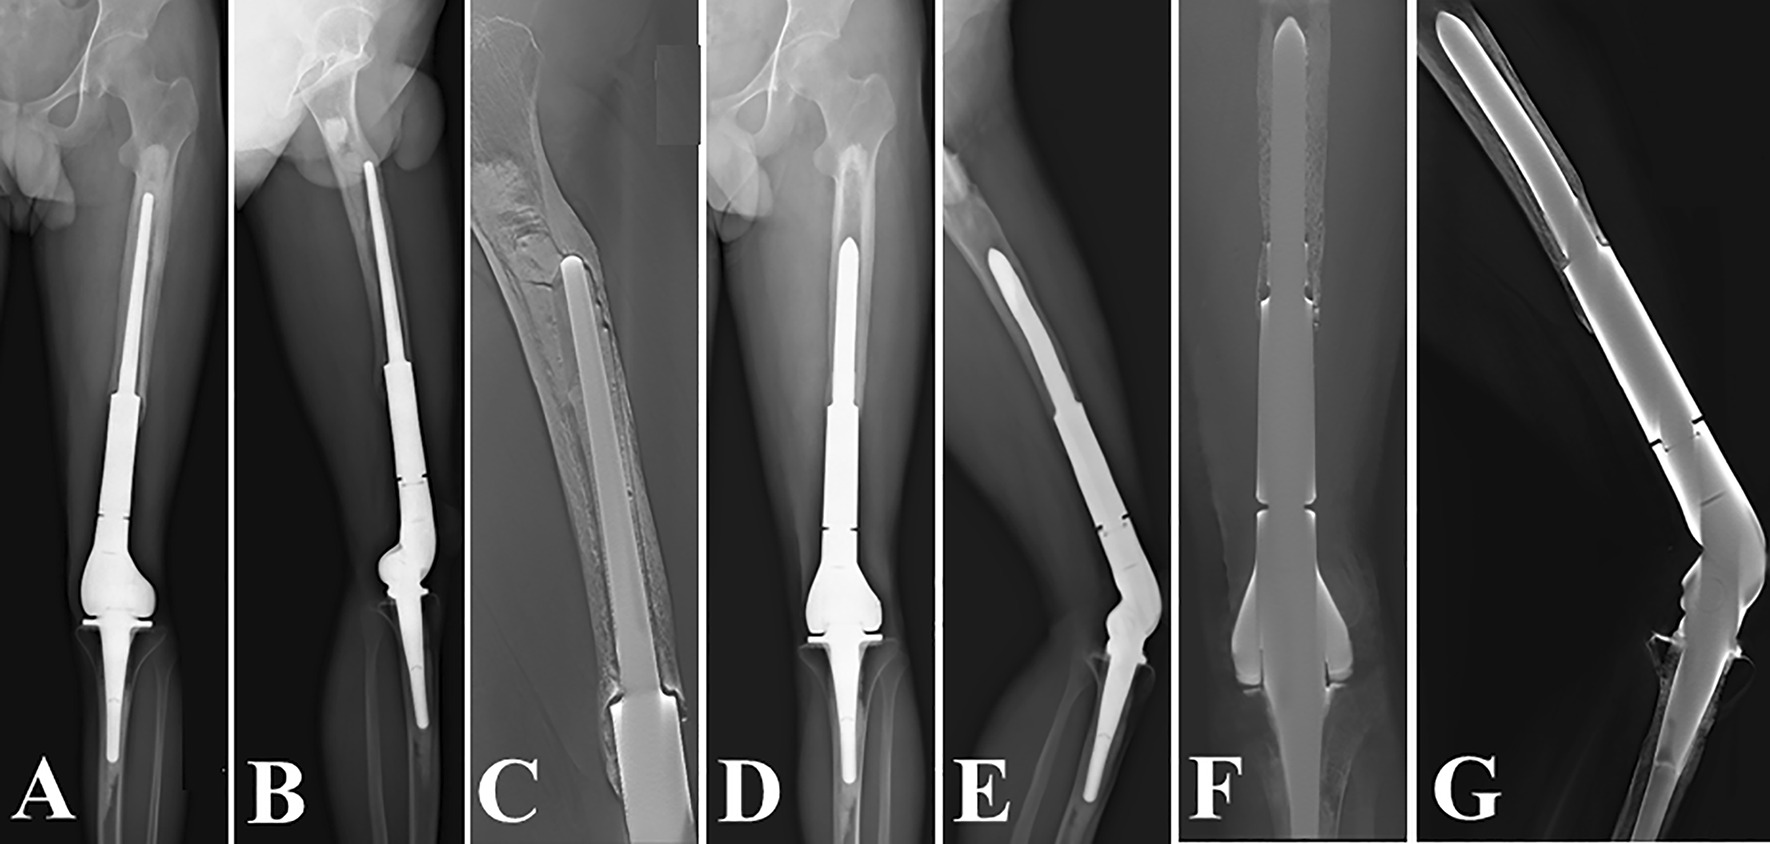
Revision of a Grade I bone loss case with “Short uncemented stem”. A–C preoperative radiographs; the anteroposterior and lateral radiographs (D, E), and the digital tomosynthesis images (F, G) 1 year after revision surgery
